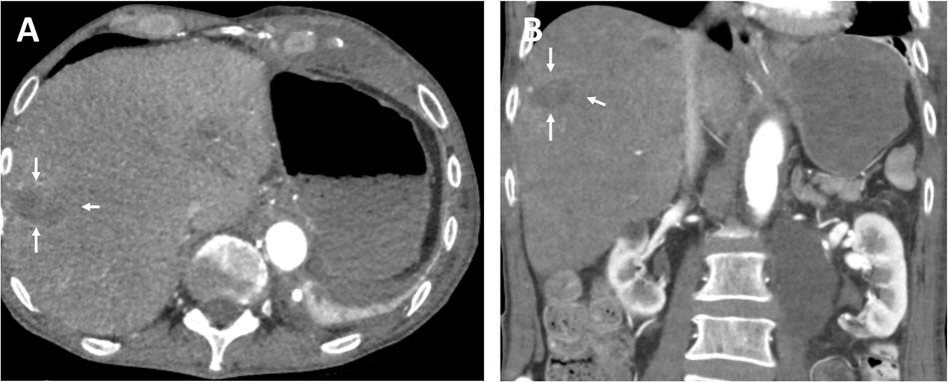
Follow-up (46 months after liver infarction) axial (A) and coronal (B) CT angiographic images demonstrate a stable hepatic infarction lesion 33 × 18 × 20 mm (arrows).
